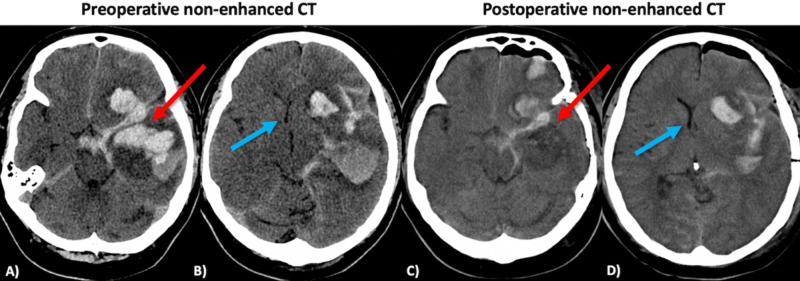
Preoperative (A,B) and postoperative (C,D) non-enhanced CT scans. A traumatic intracerebral hematoma with perifocal edema and subarachnoid hemorrhage extending into Sylvian fissure and basal cisterns was found upon the initial imaging (red arrow, slice A). The midline shift was equal to 9 mm (blue arrow, slice B). As a major part of hematoma in the temporal lobe was evacuated (red arrow, slice C), the midline shift diminished from 9 to 4 mm (blue arrow, slice D).
